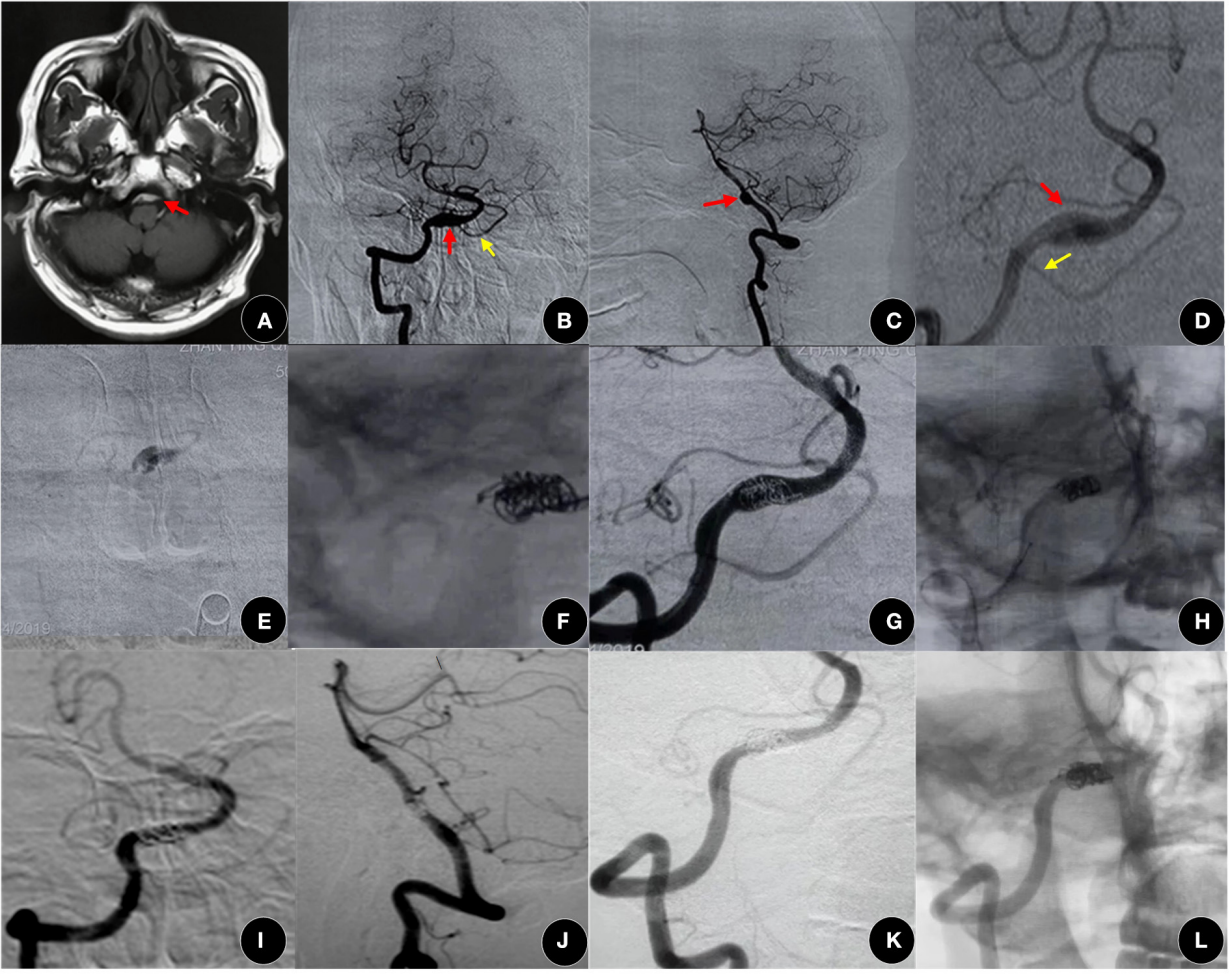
A 53-year-old man presented with SAH for 2 days. (A–D) MRI images indicated an aneurysm in the vertebrobasilar system (red arrow). (B–D) Angiographic images showed a vertebral artery dissecting aneurysm (red arrow) with a double aneurysmal cavity, located in the proximal posterior inferior cerebellar artery (yellow arrow). (E) It was confirmed that the microcatheter was in the aneurysmal cavity by the hand bolus injection contrast medium. (F) After releasing several coils, a Leo plus stent (3.5 mm × 25 mm) was deployed to press these coils evenly into the cavity, and then the other coils were continuously packed. (G,H) Good occlusion (OKM grade C) was achieved, and the VA and PICA were patented. (I–L) The embolism degree increased to complete occlusion (OKM grade D) and the modified Rankin scale (mRS) maintained a score of 0 at 13 months after surgery. VA, vertebral artery; PICA, posterior inferior cerebellar artery.
